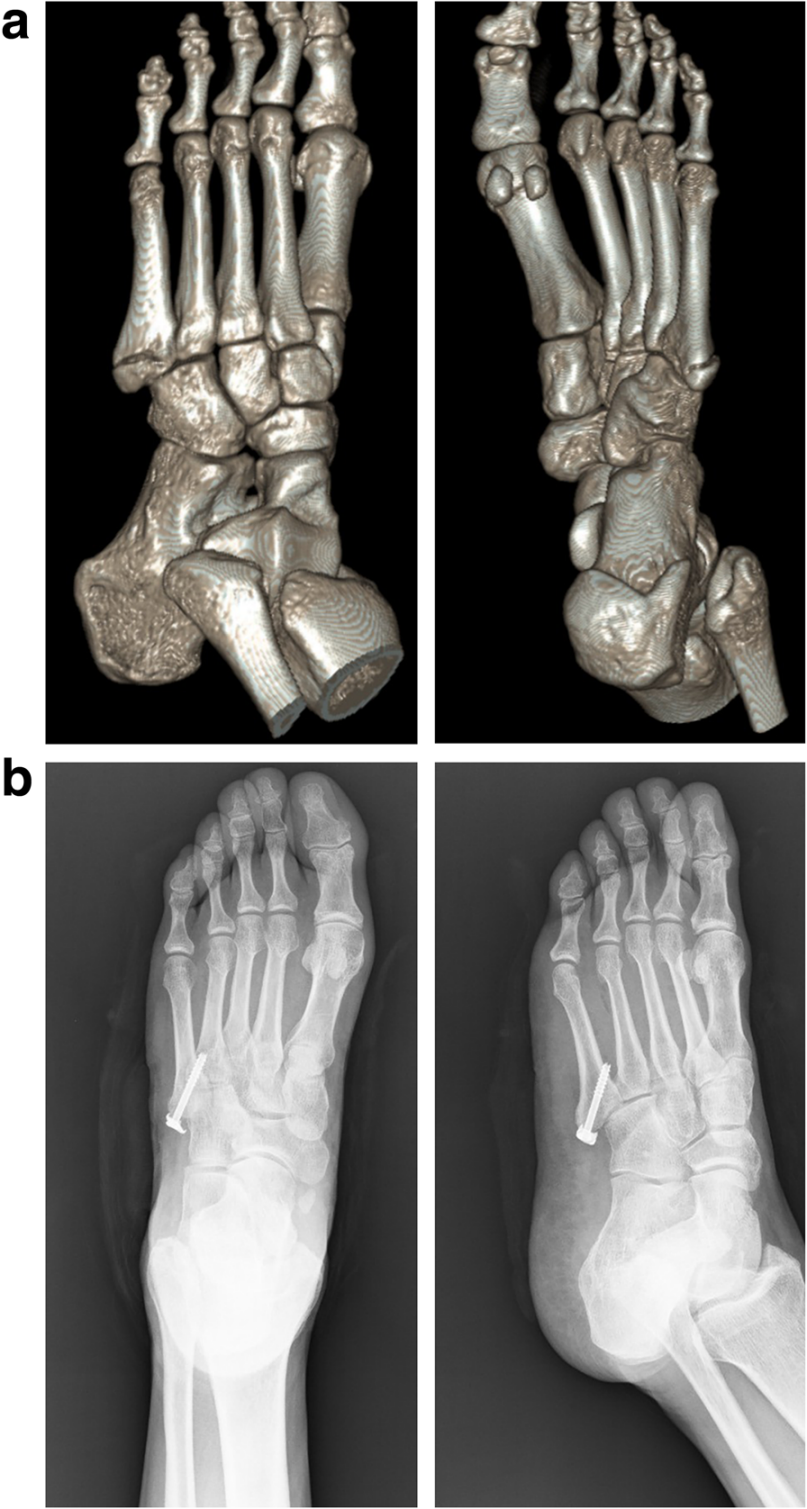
Preoperative 3D reformations of CT scans (a) and postoperative (b) plain radiographs of a fifth metatarsal base fracture that was treated surgically using the Intramedullary Screw
